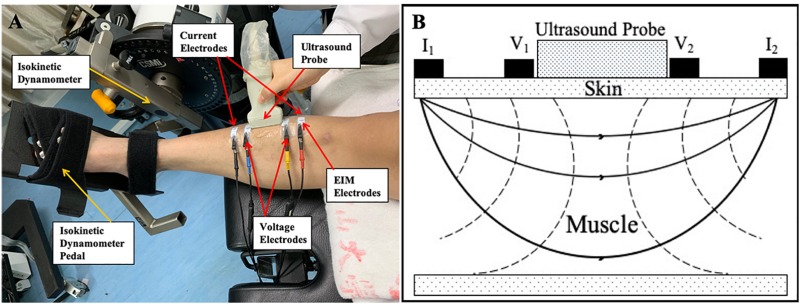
(A) One male subject was relaxed and in supine position on an instrumented bed with foot fixed on the isokinetic dynamometer pedal. One pair of current electrodes (red and black) and one pair of voltage electrodes (yellow and blue) were linearly arranged along the surface of the TA on the muscle belly. An ultrasound probe was placed between voltage current electrodes. (B) Demonstration of positions of EIM parameters and ultrasound probe. I_1_ and I_2_ were a pair of current electrodes of EIM. V_1_ and V_2_ were a pair of voltage electrodes of EIM. An ultrasound probe was placed between these two pairs of electrodes. TA, tibialis anterior; EIM, electrical impedance myography.
